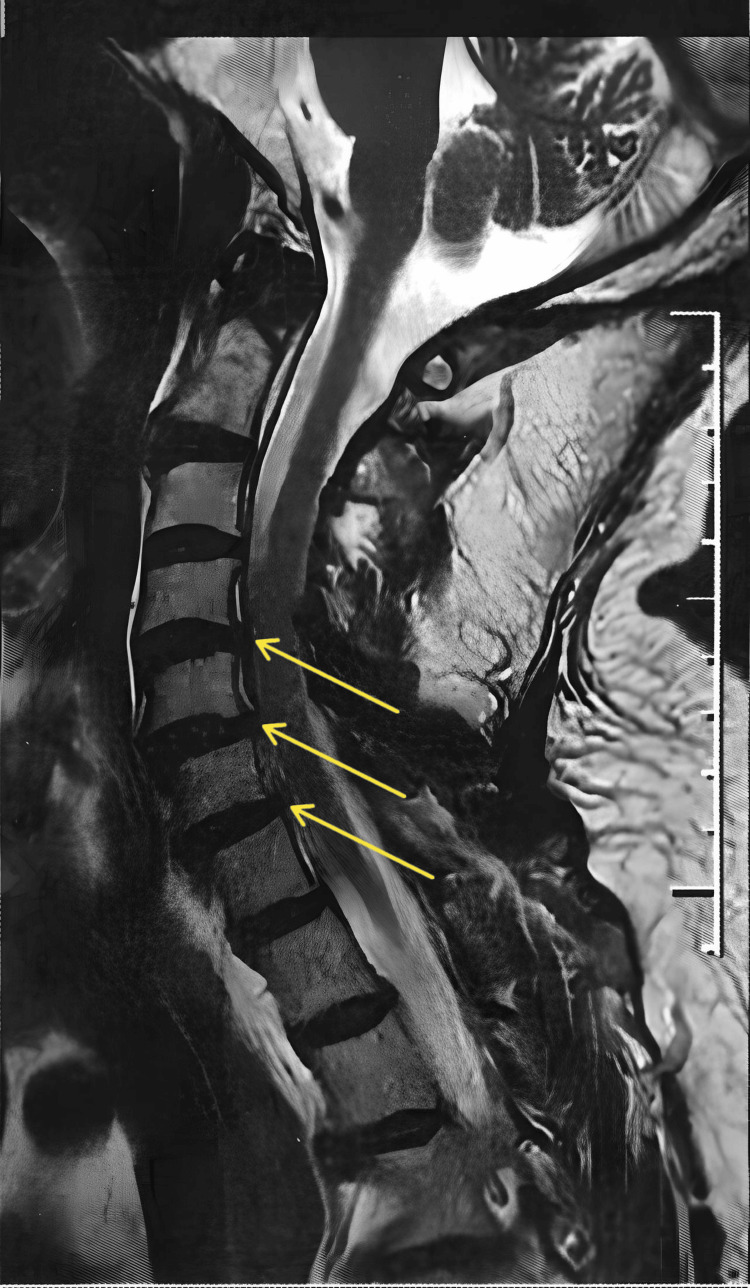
Magnetic resonance imaging of the cervical spine showing spondyloarthrosis, highlighting mild stenosis of the spinal canal at C4-C5 and C5-C6 and stenoses of the left neural foramina at C3-C4, C4-C5, C5-C6, and C6-C7
